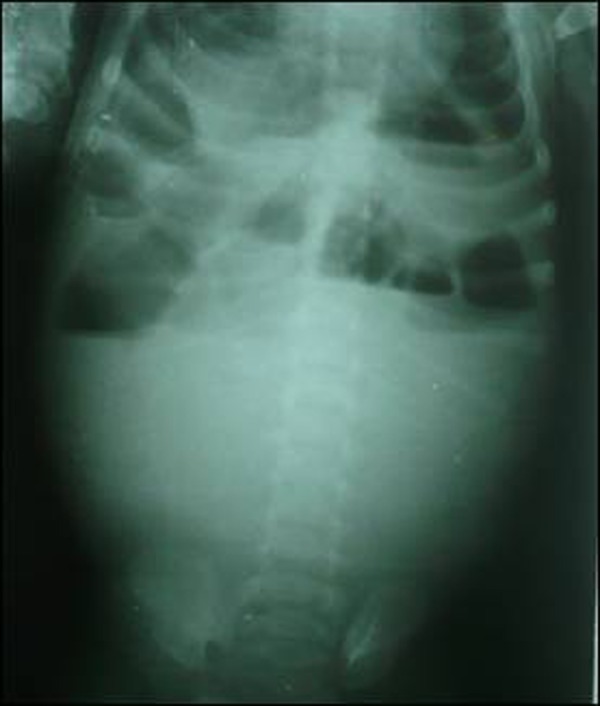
Figure 1: Abdominal radiograph suggestive of mass effect and intestinal obstruction
